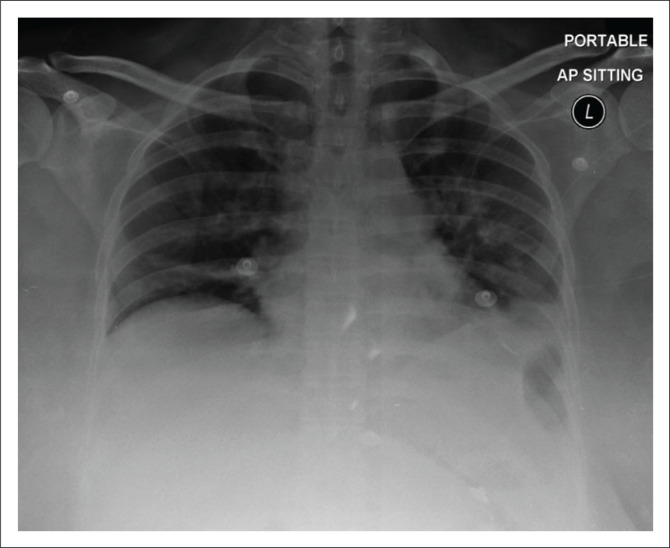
A 36-year-old female admitted to an isolation ward with COVID-19 pneumonia. Portable antero-posterior (AP) chest radiograph demonstrates blunting of the left costophrenic angle suggesting a pleural effusion as well as bilateral air space opacities. *Brixia* score: 13.
